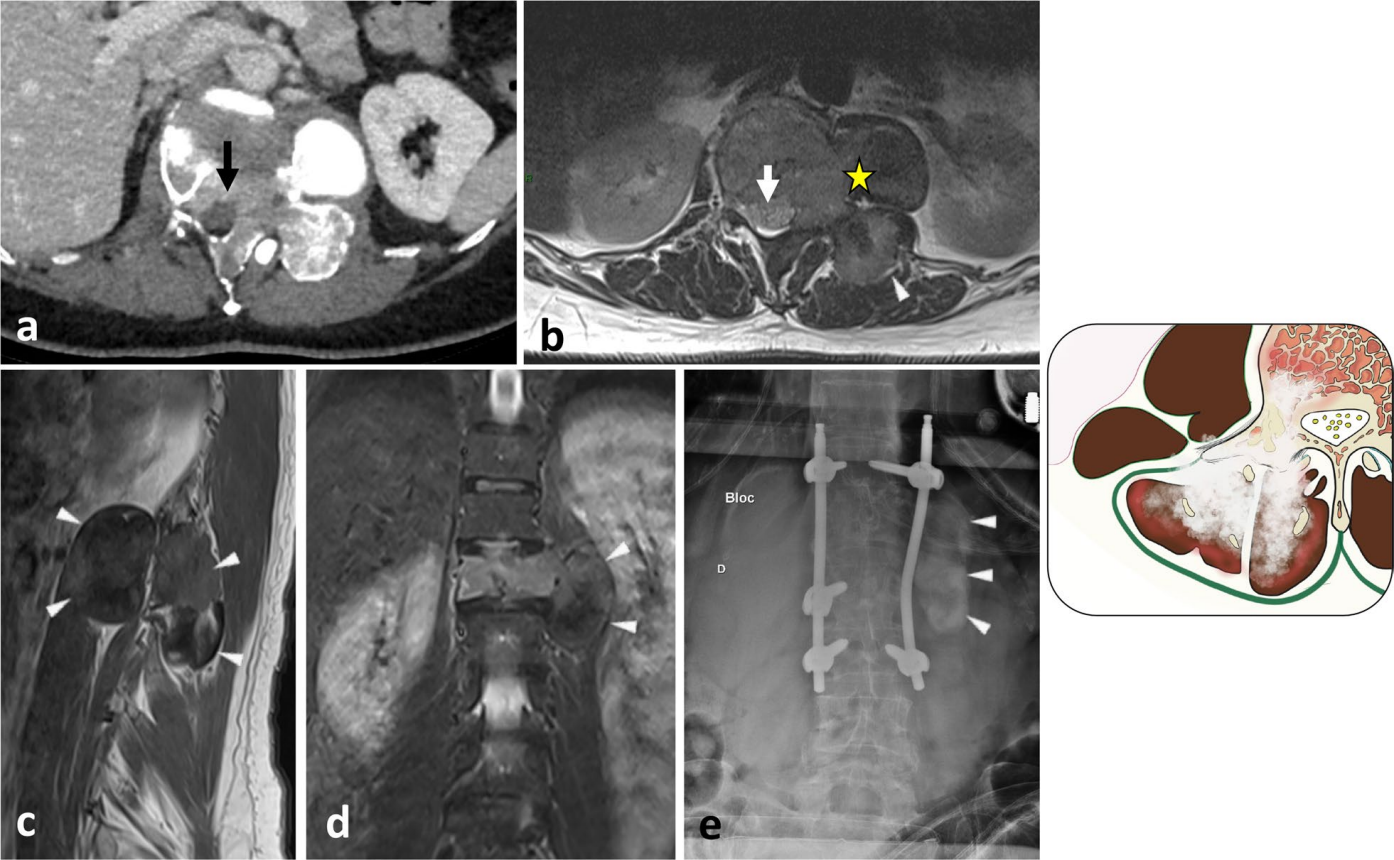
Spinal osteosarcoma* in a 23-year-old male with back pain and progressive weakness of both lower limbs. Contrast-enhanced axial CT scan reconstruction (a) shows corporeal-pedicle bone formation of L1. The presence of a fat plane between the tumour and the epaxial muscles (arrowhead) suggests that the mass turn away the epaxial muscles rather that invades them. The mass has non-specific imaging features on MRI: mild hyperintense on axial T2WI (b), hyposignal on sagittal T1WI (c), mild and heterogeneous enhancement on contrast-enhanced, fat-suppressed T1WI (d). The mass also invades epidural space (arrows). Anteroposterior radiography (e) performed after pedicle screw fixation showing bone formation in the left paraspinal region. Yellow star shows the epicentre of the mass
